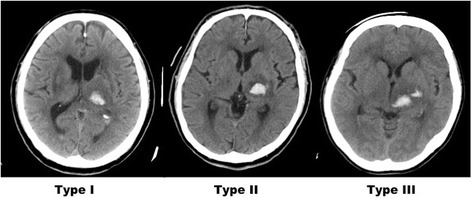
Computed tomography classification of thalamic hemorrhage. The hemorrhages were classified as follows: type I, hematoma localized in the thalamus; type II, hematoma extending into the internal capsule; and type III, hematoma extending into the midbrain
